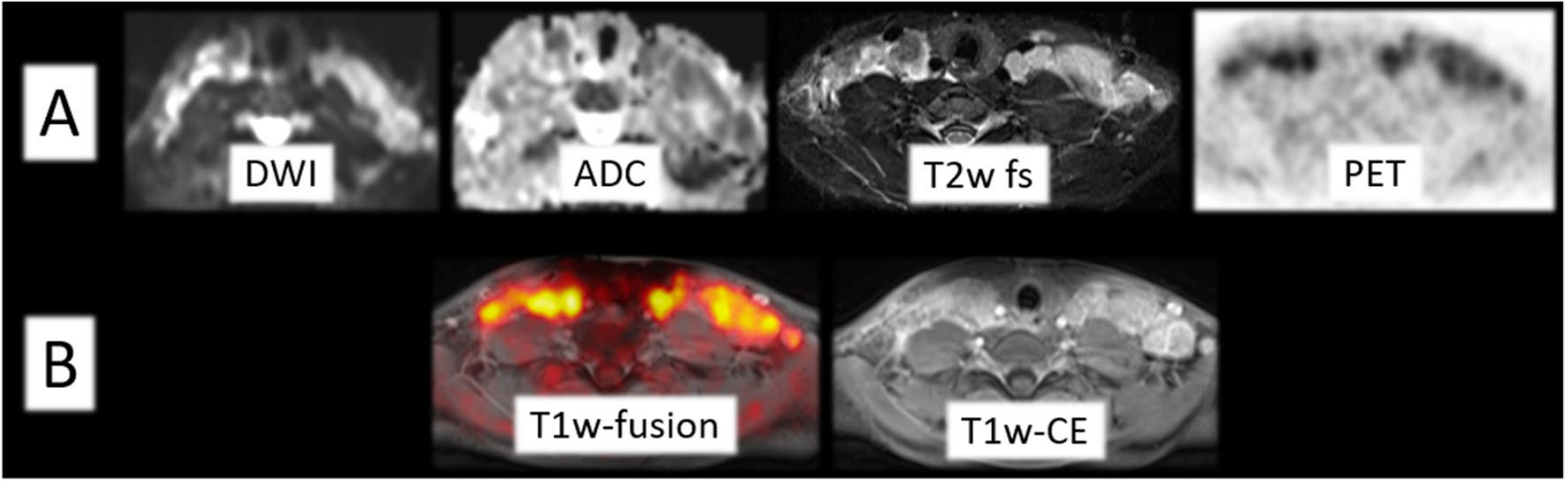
Example of a 15-year-old pediatric patient with correctly identified active lymphoma disease at PET/MRI-1 (A) and PET/MRI-2 (A, B) with visual [^18^F]FDG uptake (SUVmax: 13.1). No additional diagnostic benefit of the T1-weighted (w) contrast-enhanced (CE) sequence was seen
